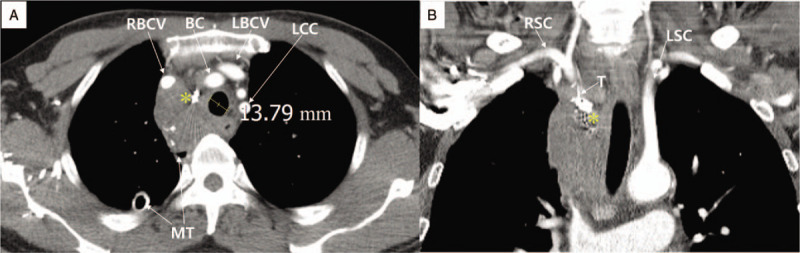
Chest computed tomography. A. The 1-week image after coronary stenting shows the decreased amount of mediastinal hematoma and the increased tracheal diameter. B. The 1-week coronal section image after coronary stenting shows the occluded terminal branch of the right inferior thyroid artery, with endovascular embolization using a microcoil and glue but the mediastinal hematoma remained. An asterisk indicates the Tornado microcoils and glue remnants applied to the bleeding vessels. BC = brachiocephalic artery, LBCV = left brachiocephalic vein, LCC = left common carotid artery, LSC = left subclavian artery, MT = mediastinal drainage tube, RBCV = right brachiocephalic vein, RCS = right subclavian artery, T = right inferior thyroid artery.
